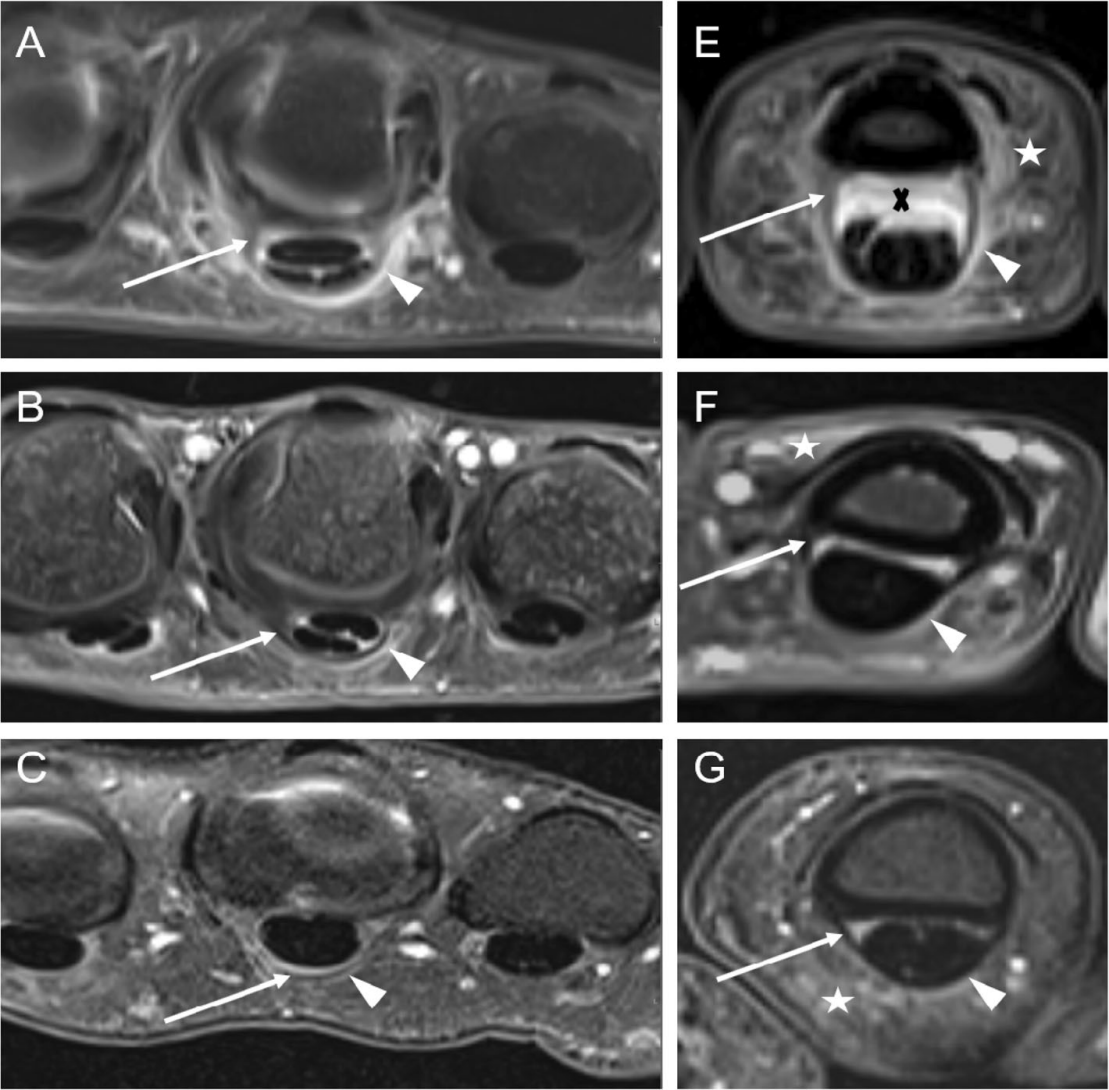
High-resolution MRI of the Hand - Figure 2
